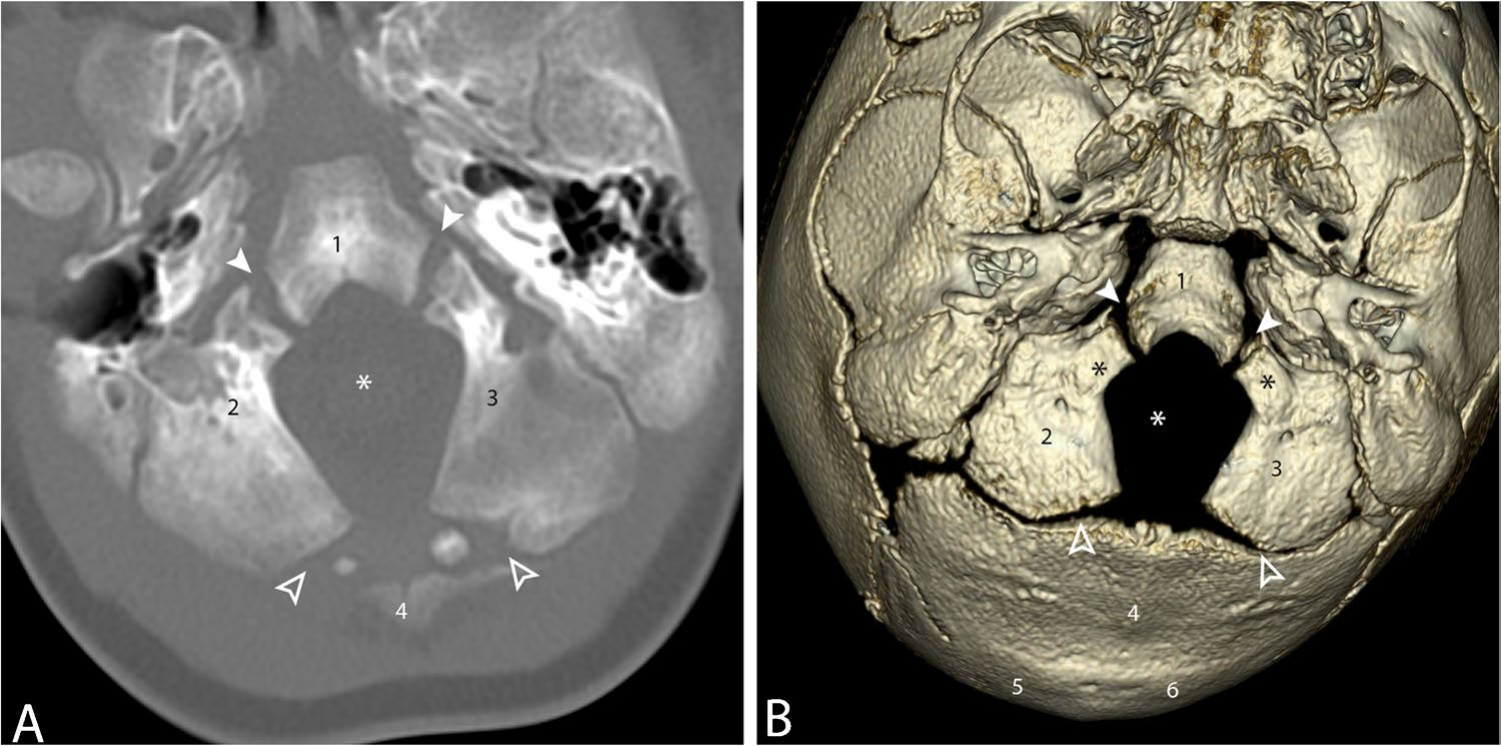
A Axial CT through the occipital bone in a 15-month-old male. B Inferior view of a 3-D reformation of the occipital bone. There are six ossification centers of the occipital bone. Three develop by enchondral ossification and three by membranous ossification. The three enchondral ossification centers (labeled with *black numerals*) are basiocciput (*1*), the caudal aspect of the clivus; right (*2*) and left (*3*) exoccipital bones, which include the occipital condyles (*black asterisks*). The three membranous ossification centers (labeled with *white numerals*) are supraoccipital (*4*), of which the opisthion is a part, and right (*5*) and left (*6*) interparietal. Note the foramen magnum, denoted by the *white asterisk*, is circumscribed completely by components of the occipital bone. The *white arrowheads* denote the ventral intraoccipital synchondroses and the *hollow arrowheads* denote the posterior intraoccipital synchondroses
